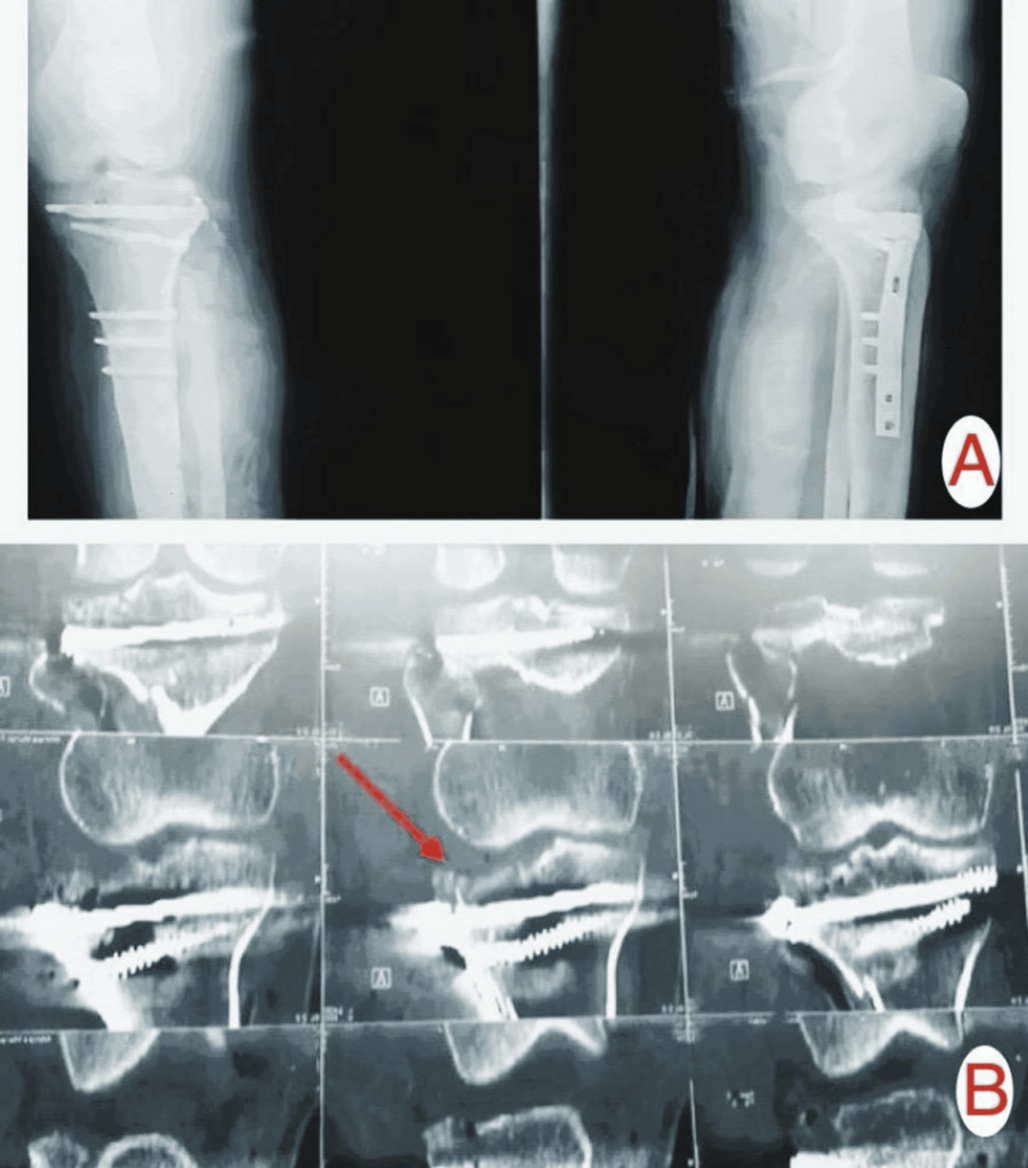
Six-month follow-up (A) X-ray, and (B) CT scan images of Patient B showing secondary depression of the reduced lateral tibial plateau articular surface
